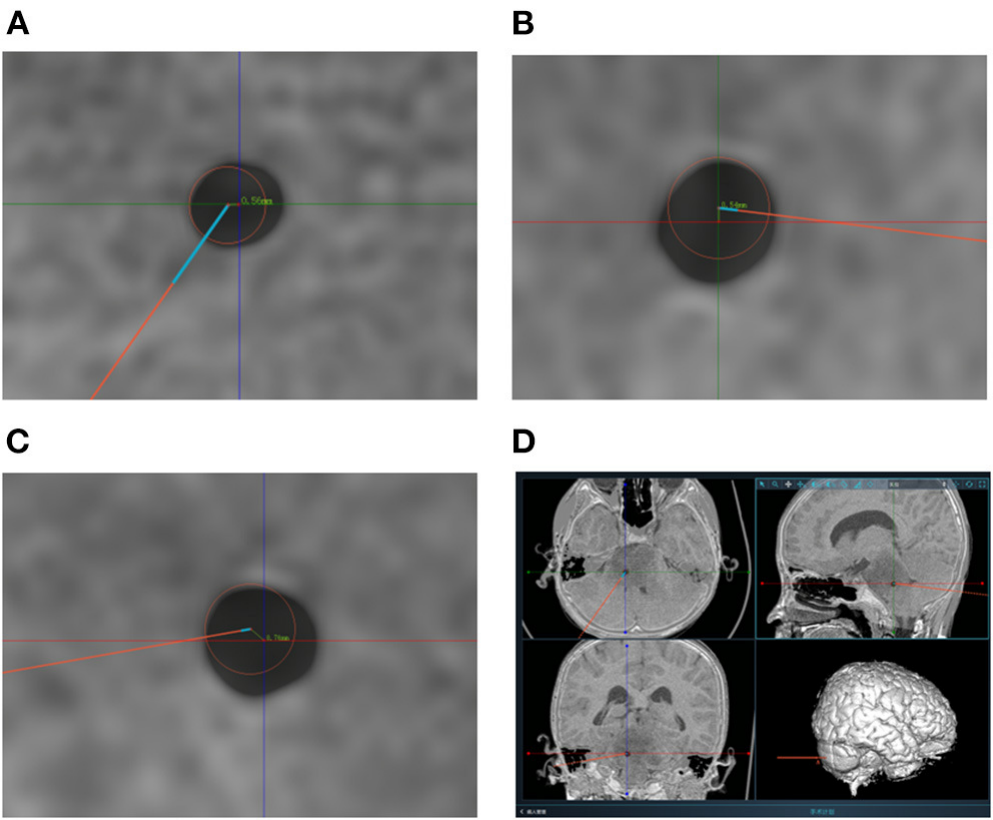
(A–C) Measurement of target point error based on postoperative CT scanning. The centers of the red circles represent the target points planned preoperatively. The TPEs are computed based on the errors from axial position, coronal position and sagittal position respectively. (D) The picture shows the measurement of one of the patients. And the TPEs were 0.52, 0.54, and 0.76 mm, respectively. The actual TPE was 1.07 mm.
